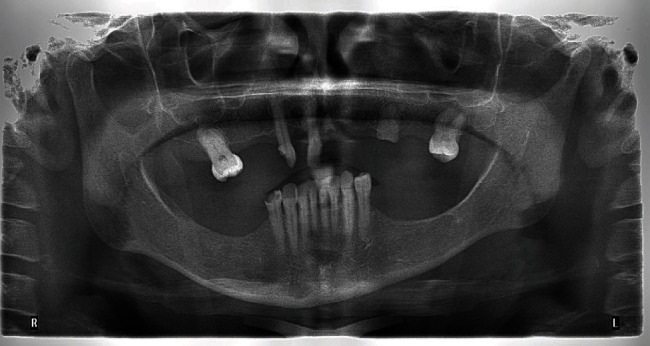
Orthopantomogram radiograph.
